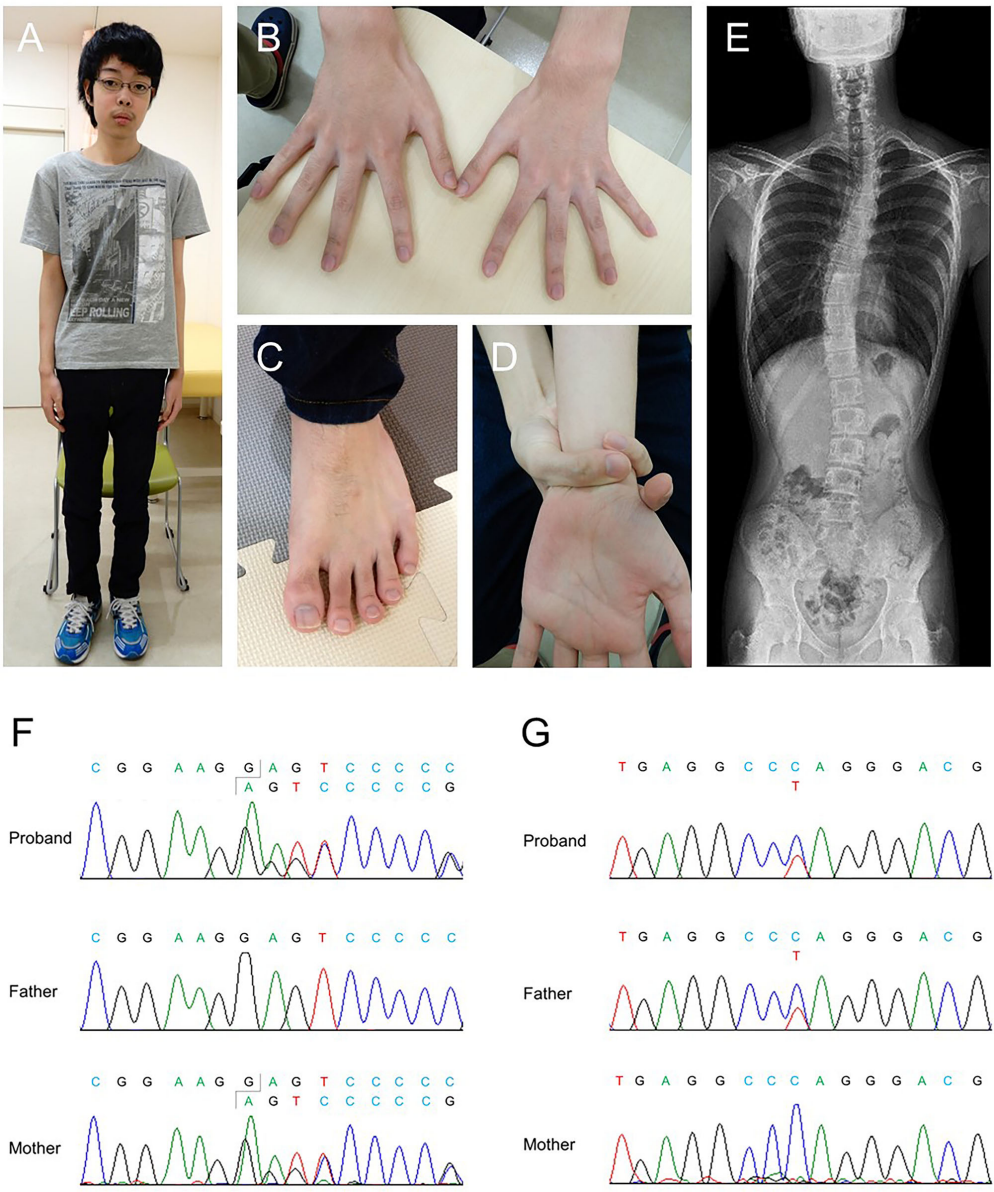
Clinical and molecular findings of the present case. A–E, Whole body (A), hands (B), foot (C) and positive wrist sign (D) photographs and a spine radiograph (E) of the proband at the age of 15 years. F, G, Electropherograms of Sanger sequencing revealing compound heterozygous *CPAMD8* variants of c.2801delG (F) and c.4552C>T (G).
